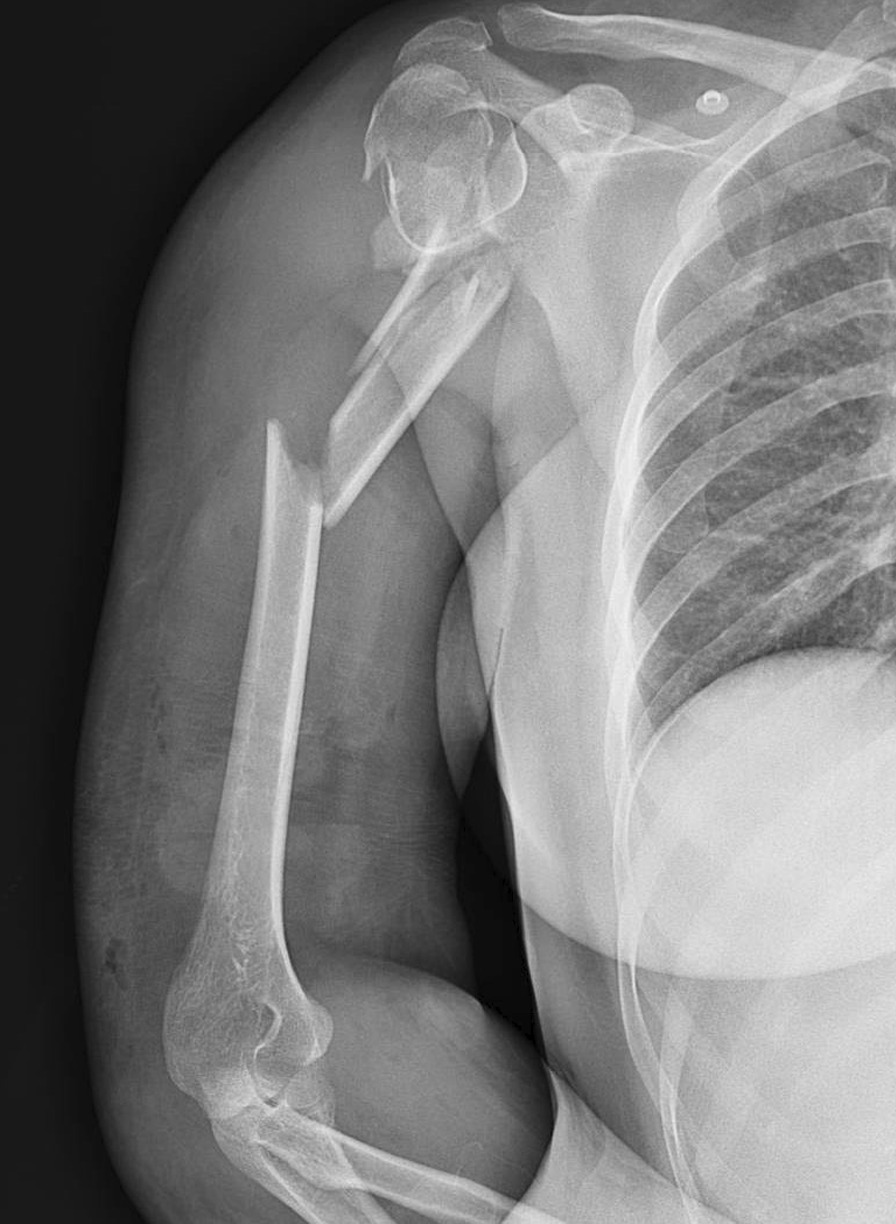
Concomitant 3-part head-splitting proximal humerus and comminuted humeral shaft fractures in preoperative X-ray
